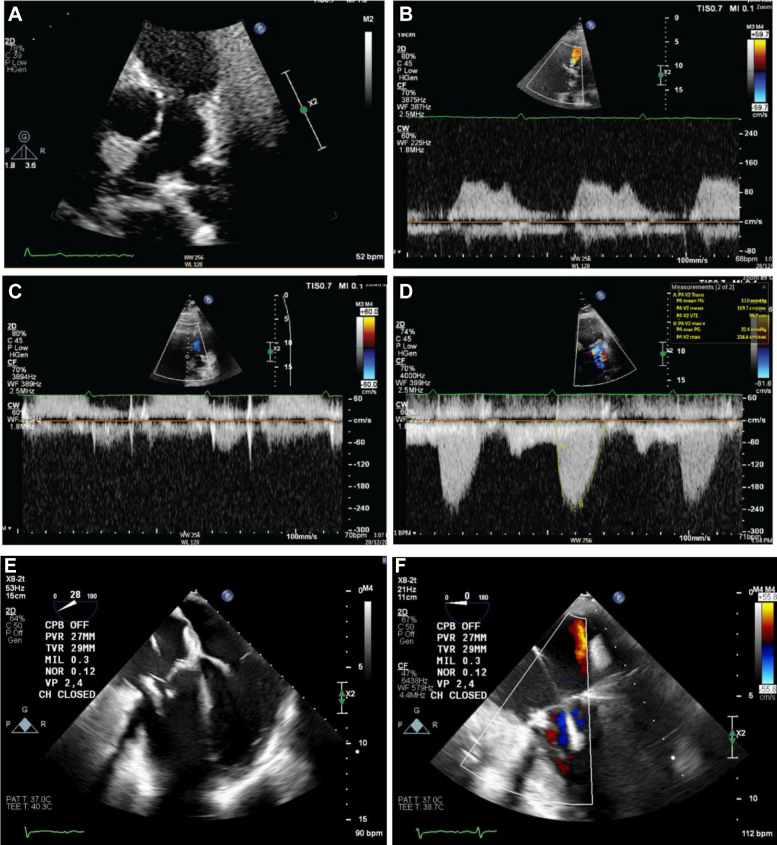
Post-Operative TOE and TTE (A) Intraoperative TOE demonstrating satisfactory pulmonary valve replacement positioning. (B) Postoperative TTE demonstrating tricuspid inflow. (C) Postoperative TTE demonstrating abolition of significant TR gradient (TV mean PG 4 mm Hg). (D) Postoperative TTE demonstrating reduced pulmonary transvalvular gradients (PV max PG 13 mm Hg, mean PG 7 mm Hg). (E) Intraoperative TOE demonstrating satisfactory tricuspid valve replacement positioning. (F) Abolition of significant tricuspid regurgitation as demonstrated on postoperative TOE with color Doppler. PG = pressure gradient; PV = pulmonary valve; TOE = transesophageal echocardiography; TR = tricuspid regurgitation; TTE = transthoracic echocardiography; TV = tricuspid valve.
